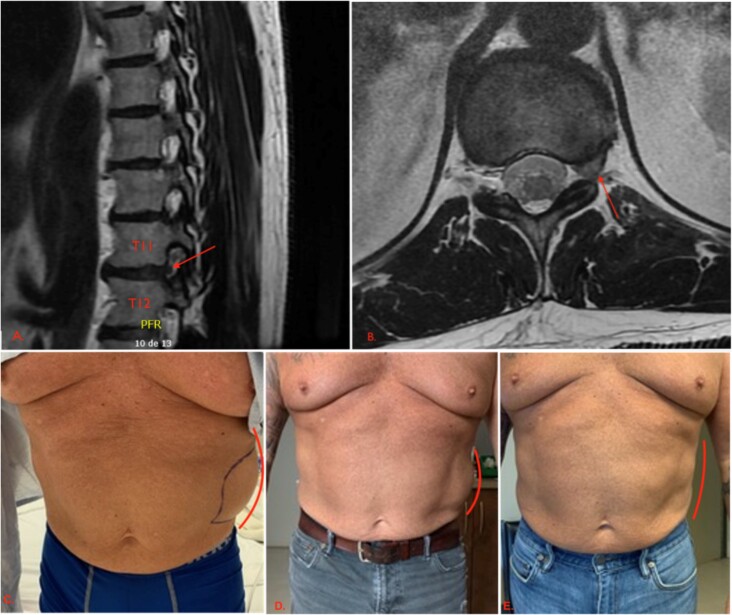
First patient’s initial presentation and clinical evolution. (A) An axial view of a CT scan of the abdomen and pelvis revealing a left-sided flank bulge consistent with the patient’s complaint without evidence of hernia. (B, C, D) Left flank bulge at, respectively, 1, 3, and 10 months follow-up, showing a progressive reduction in size (lines). The pictures were given with the patient’s consent.
